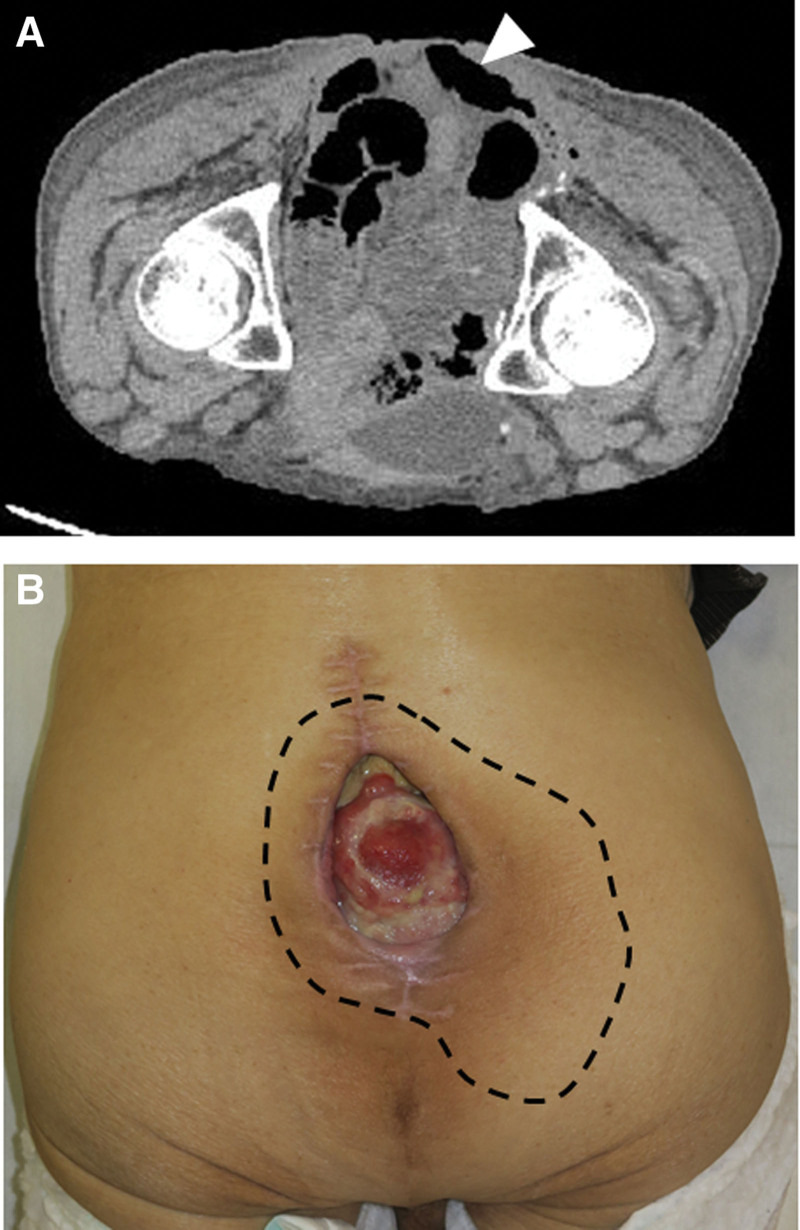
(a) Patient’s wound at first visit to outpatient clinic of plastic surgery department. After resection of the rectus and part of the pelvis, the surgical scar showed dehiscence and the retroperitoneum was exposed. The size of the ulcer was 5.5 × 4.5 cm and it had a pocket width of 16 × 12 cm (area surrounded by the dotted line); (b) Computed tomography image of the wound recorded with patient in the prone position, prior to surgery. The retroperitoneum is exposed (outside the body), and the intestinal tract is located directly below the wound (arrowhead).
